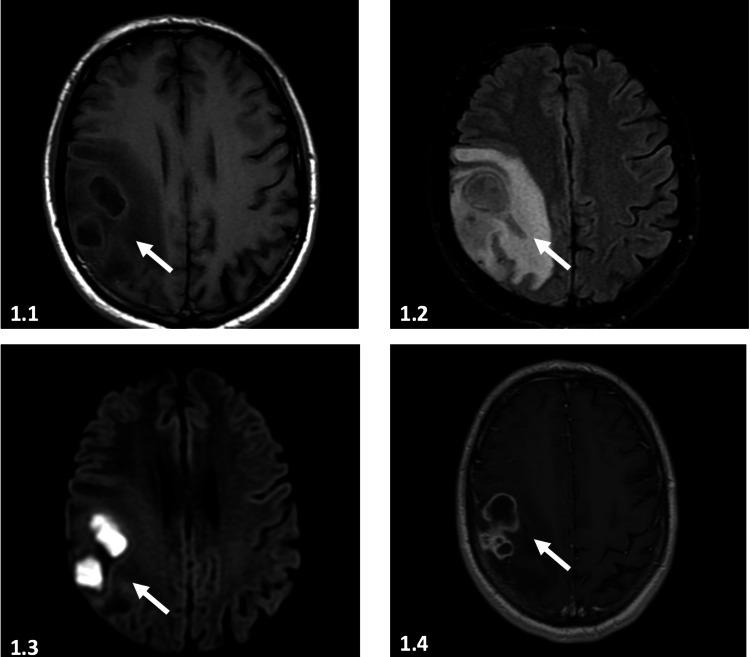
Brain MRI A) T1 sequence: space-occupying process, hypointense with isointense wall and significant vasogenic edema located in the right precentral gyrus. B) T2 FLAIR sequence: lesion with double wall image that is hyperintense in its internal wall and isointense in its external wall with vasogenic edema, obliteration of the subarachnoid space. C) Diffusion sequence: significant and homogeneous increase in the signal intensity of the central area of ​​the lesion. D) Contrast-enhancedT1 sequence: annular, intense reinforcement with an irregular but well-defined contour of its wall.
